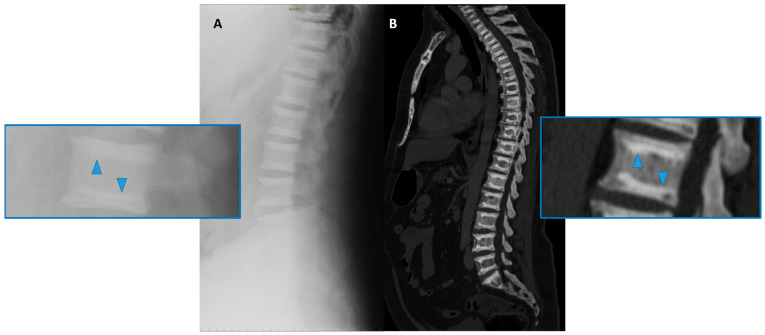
Conventional radiography (lateral projection, (A)) and Computed Tomography (CT Sagittal reconstruction, (B)) of the spine of a 50-year-old male affected by osteopetrosis (ADO type 2). Well-defined areas of increased bone density are detectable on the vertebral endplates (arrowhead), configuring the so-called ‘sandwich vertebrae’ appearance.
